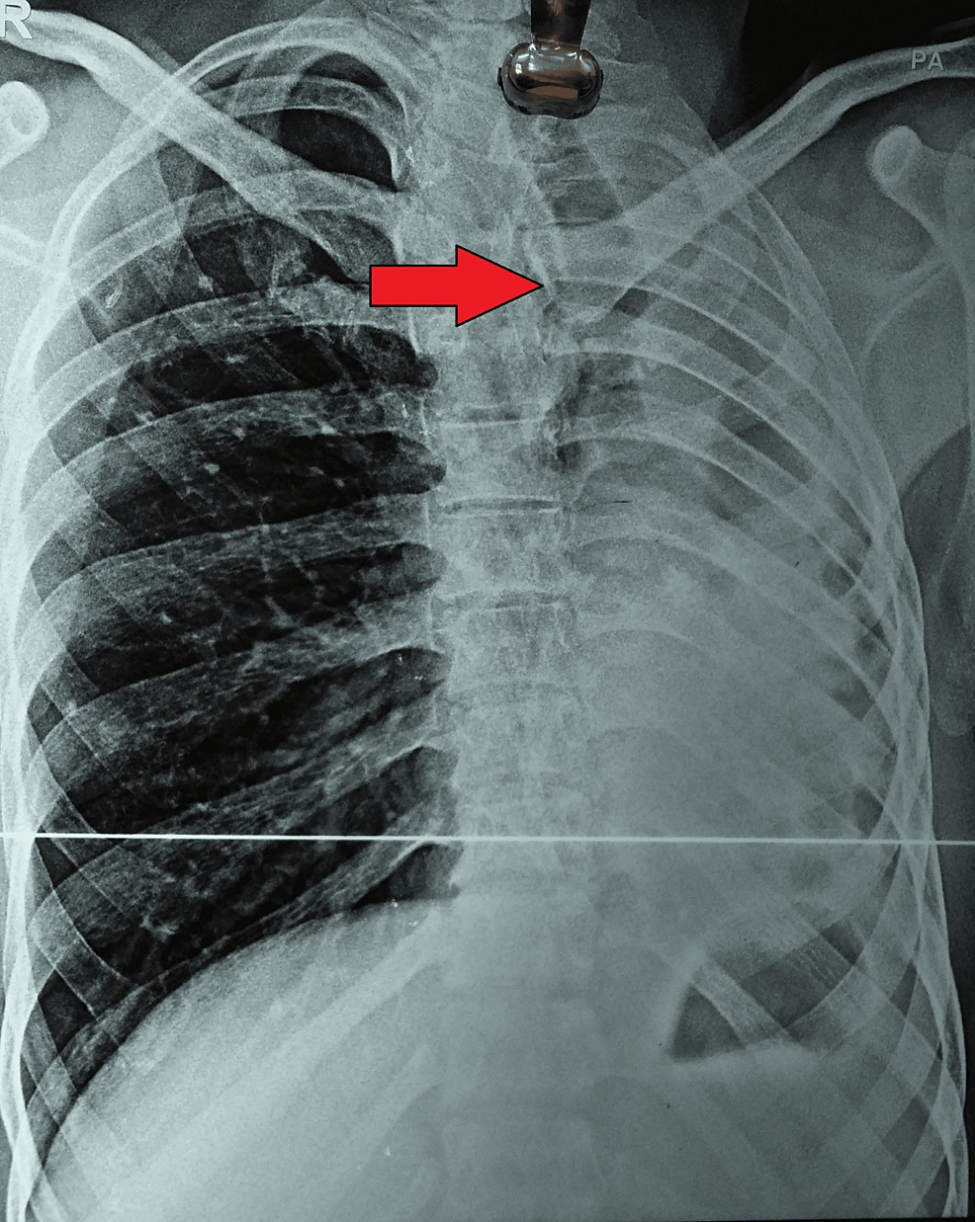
Chest radiograph (posteroanterior) view showing left lung involvement
